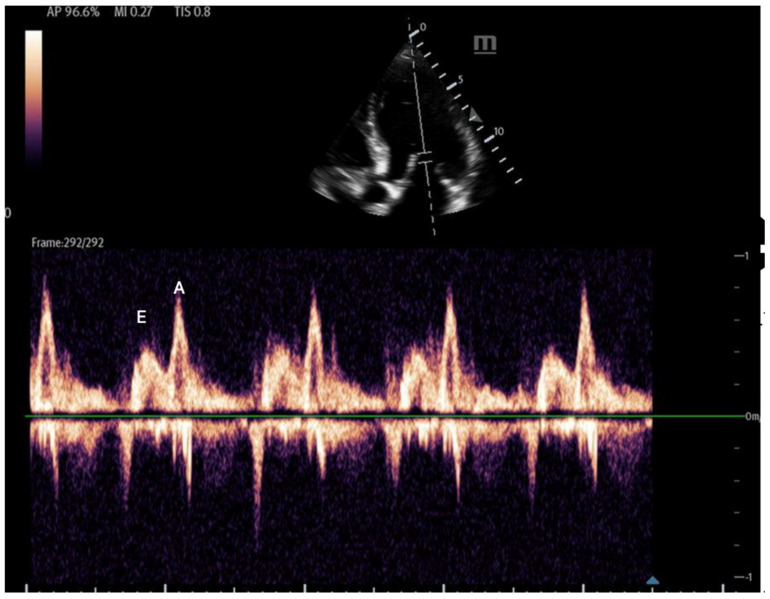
Mitral inflow with PW Doppler gate at mitral valve tip (E and A waves).
